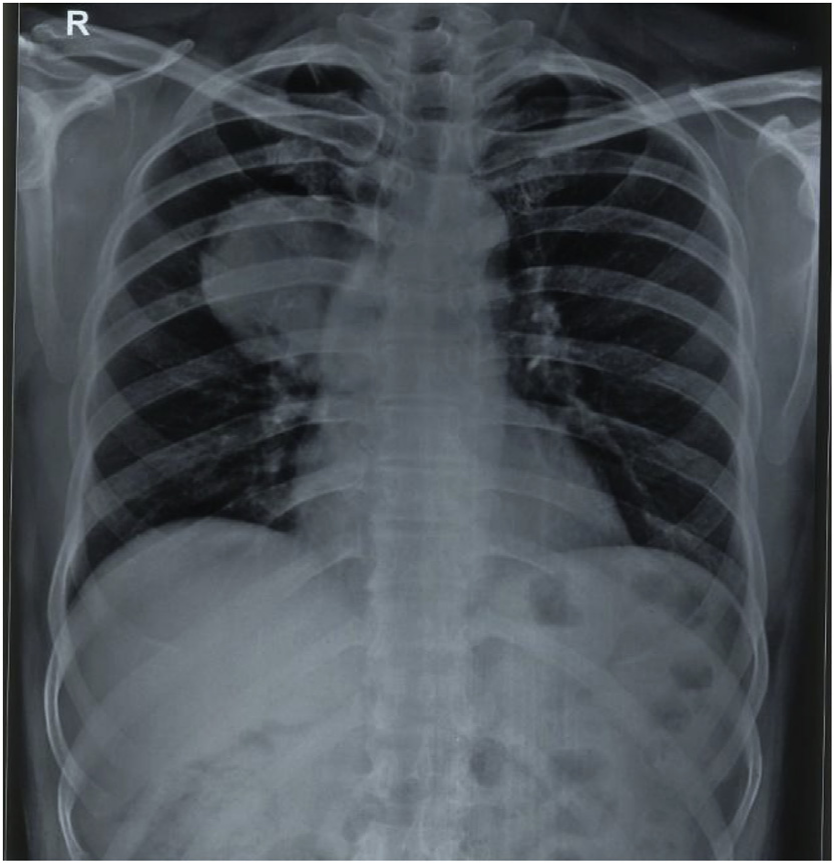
Chest x-ray showing growth in right upper lobe.
